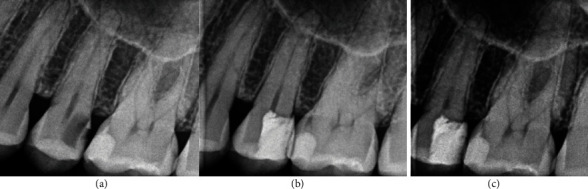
Permanent tooth 25. The image (a) shows diagnostic X-ray, image (b) immediate postoperative, and image (c) control at 24 months.
